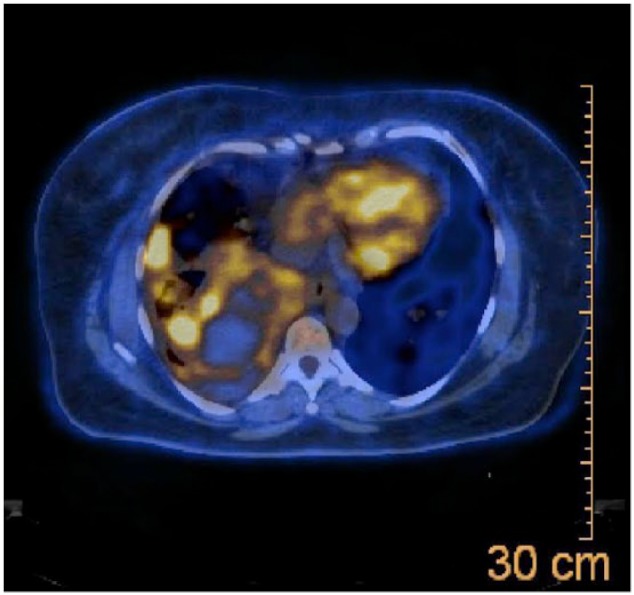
Positron emission tomography–computed tomography scan of chest showing multiple pulmonary nodules.
